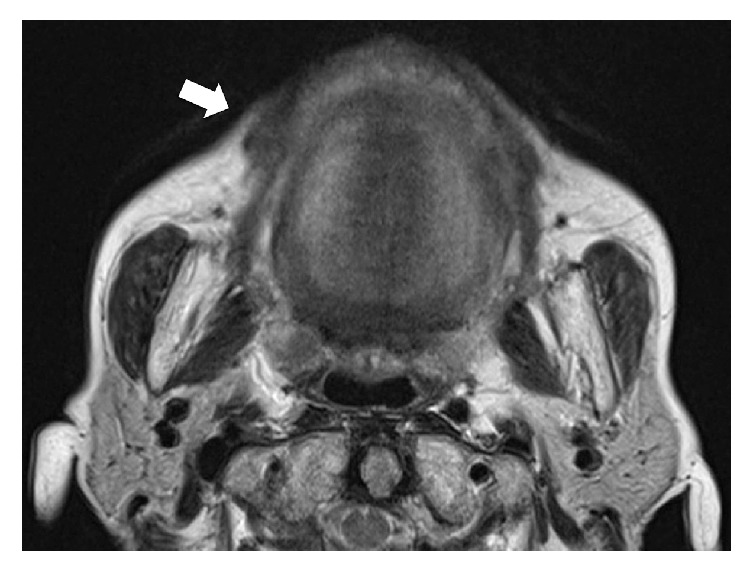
MR image of the lesion (arrow).
